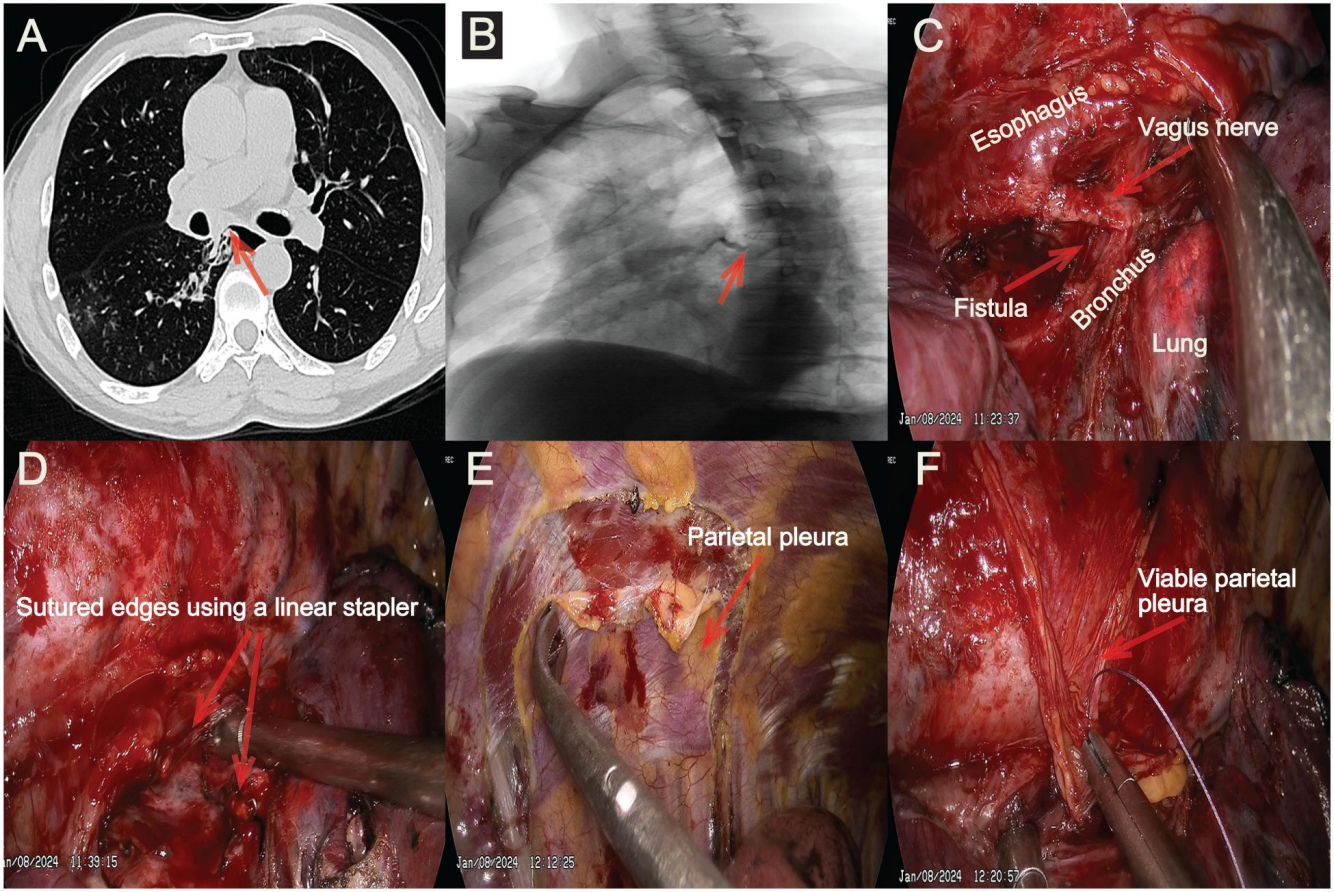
(A) Computed tomography revealed chronic right lower pneumonia and the abnormal tract (arrow). (B) Videofluoroscopy confirmed the fistula connecting the oesophagus and the right middle bronchus (arrow). (C) Identification and exposure of the anomalous fistula. (D) After being incised and sutured using a linear stapler. (E) Plan the pleural area based on the scope. (F) Using a piece of viable parietal pleura to interpose between oesophageal and bronchial closures
